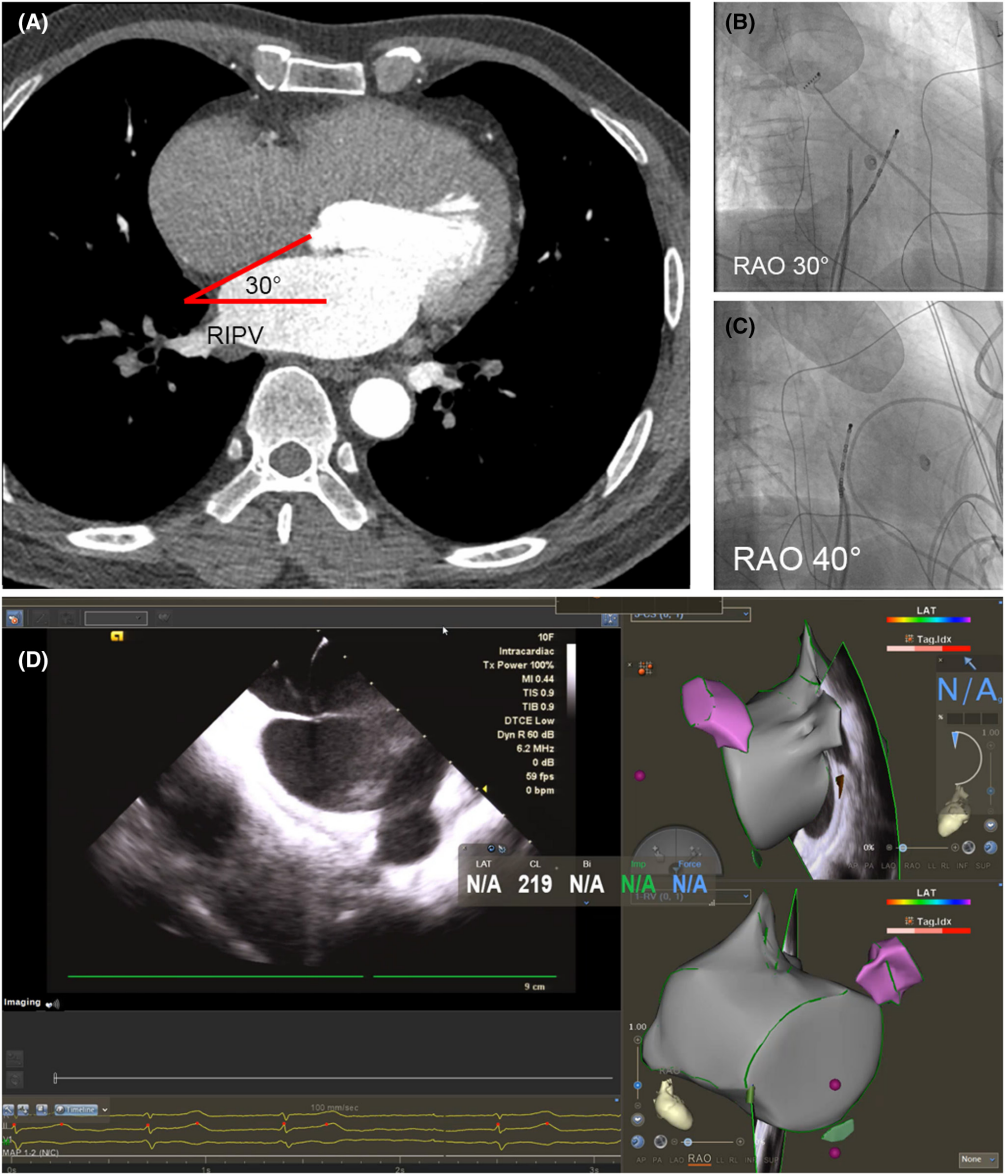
An example of transseptal puncture guided by multidetector computed tomography (MDCT) and verified by intracardiac echo in the index cohort. (A) The orientation of IAS was 30° measured from MDCT scan. (B, C) The tip of sheath pointed to 12 o'clock in the X‐ray image from RAO 30°, while pointed to 11 o'clock in traditional RAO 40°. (D) Intracardiac echo visualized the left upper pulmonary vein and fossa ovalis (FO), with “tenting” within the middle of the FO.
